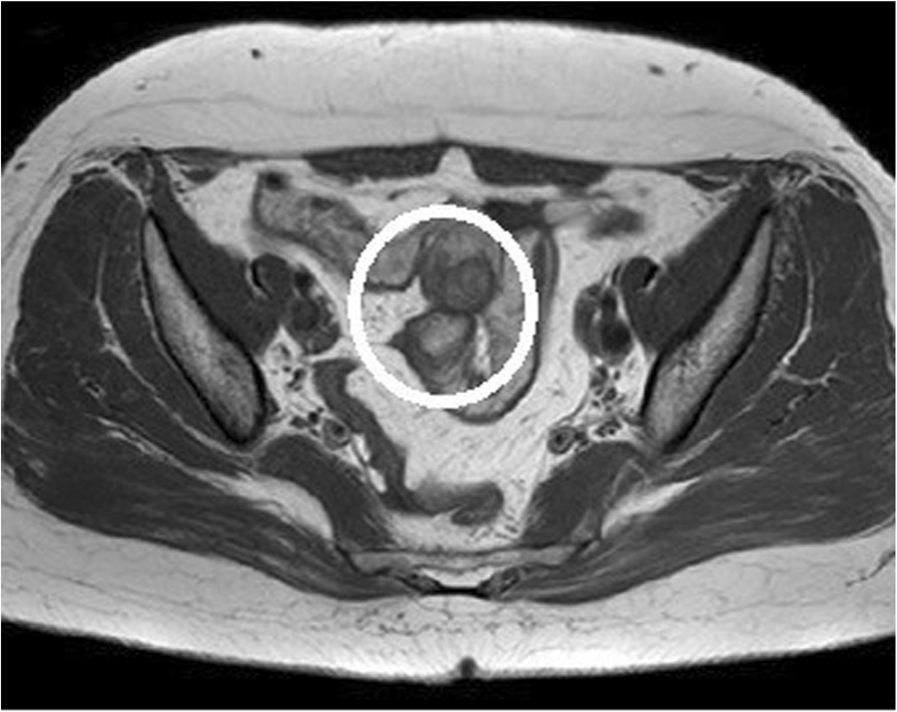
MRI shows a mass at the end of the ileum (circle)
